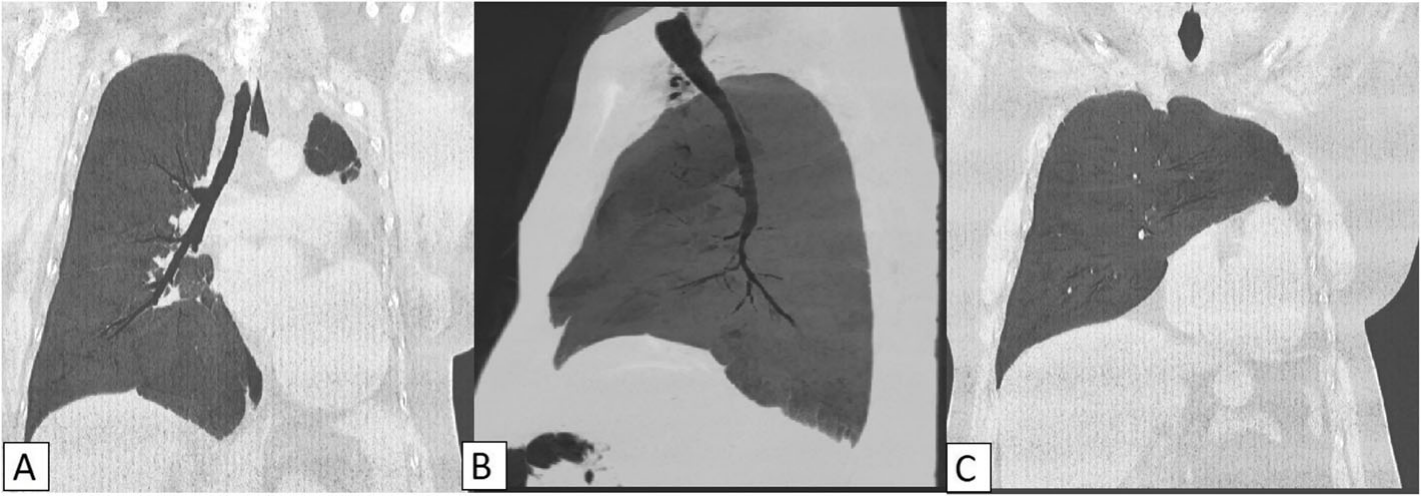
Minimum intensity projection CT images in coronal and sagittal planes show solitary right bronchus and lung (A, B). Mild tracheal narrowing is seen in its upper intrathoracic part (B). There is herniation of hypertrophied right lung across the midline into the left upper hemithorax (C)
